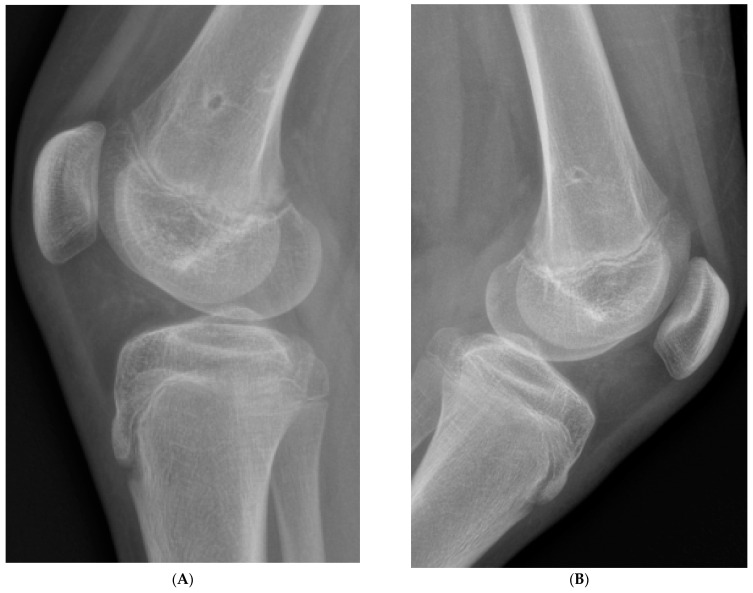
(A,B) depict lateral radiographs of the knees of two different children, each showing an open growth plate and visible holes from previously placed (and now removed) metaphyseal screws. On follow-up, the holes in both cases have shifted further away from the growth plate, indicating that bone growth remains active in each child.
